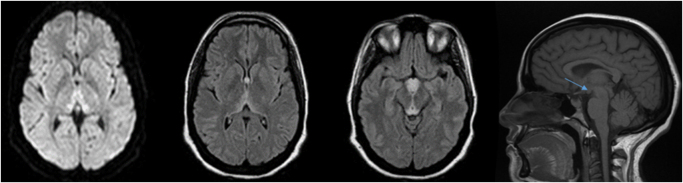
Brain MRI (axial and sagittal views, Diffusion, FLAIR and T1 sequences) showing bilateral hyperintensities in the medial thalami, mammillary bodies, and periaqueductal region.
